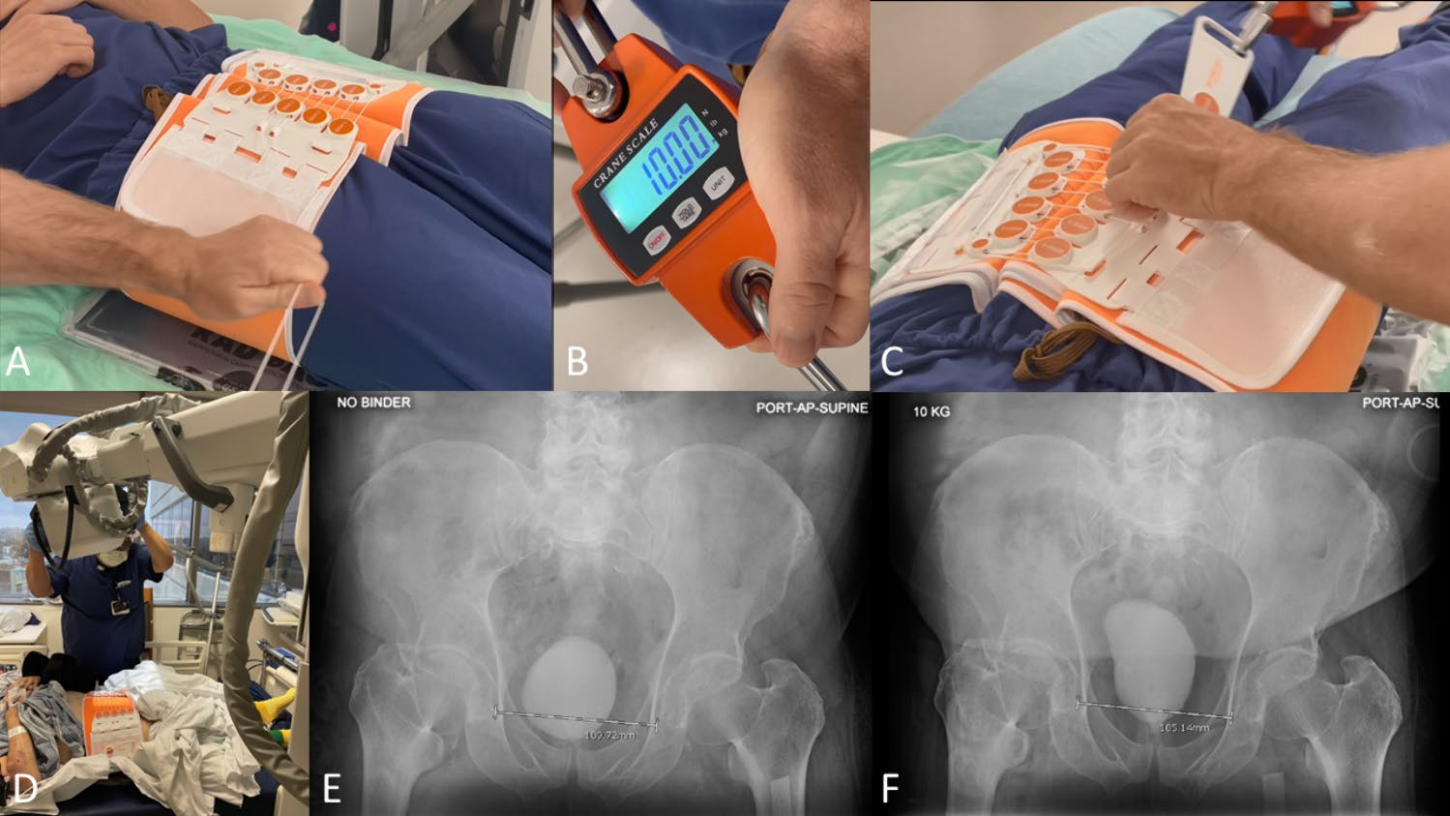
Photographs of surgeon (A) tensioning of a pelvic binder (B) using a hanging scale to a standardized force of 10 kg and (C) securing the binder in a compressed position. (D) Photograph of a 91-year-old-man with a minimally displaced LC1 fragility fracture of the pelvis comfortably undergoing pelvic binder radiography at 10 kg. (C) Non-stress and (D) stress radiographs of this patient’s fracture demonstrating 4 mm of displacement at 10 kg
